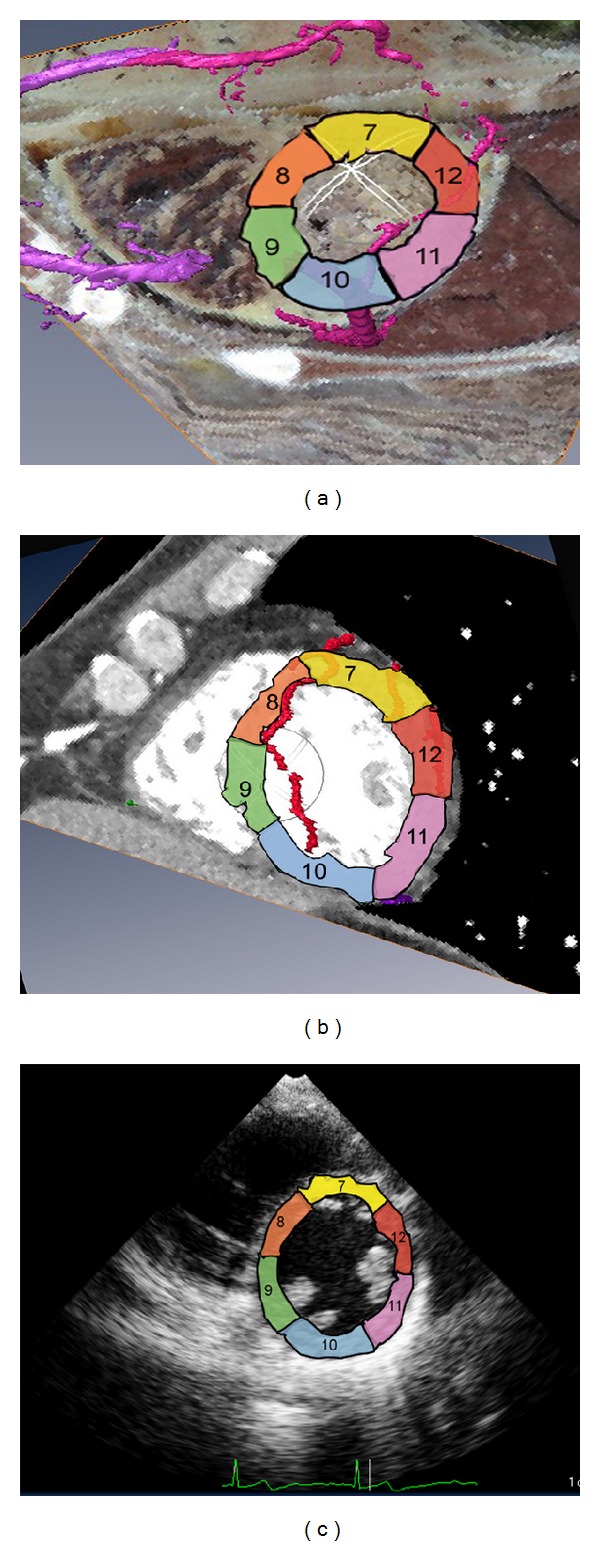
Short-axis sections of the left ventricle at the level of the papillary muscle. (a) Fusion display of the CVH dataset and the coronary artery model. (b) Fusion display of the cardiac CT dataset and the coronary artery model. (c) Short-axis section of the left ventricle at the level of the papillary muscle revealed by echocardiography.
